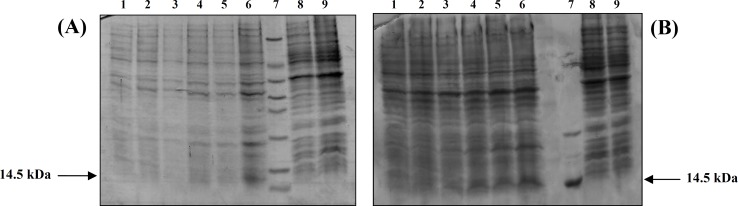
Comparison of SDS-PAGE analysis of mature and mutated recombinant CTB in *E. coli*. A) SDS-PAGE analysis of expression of mature recombinant CTB in *E. coli* M15 (pREP4) using pQE_*ctx*B as expression vector. Lane 1, M15 (pREP4) containing pQE_*ctx*B before induction with IPTG; lane 2-6, indicate 1-5 hours after induction respectively; lane 7, protein size marker and lane 8 and 9, M15 (pREP4) containing pQE used as negative control. B) SDS-PAGE analysis of expression of mutant recombinant CTB in *E. coli* M15 (pREP4using pQE containing mutant *ctx*B gene as expression vector. Lane 1, M15 (pREP4) containing pQE_*ctx*B before induction with IPTG; lane 2-6, indicate 1-5 hours after induction respectively; lane 7, commercial CT (cholera toxin) used as positive control and lane 8 and 9, M15 (pREP4) containing pQE used as negative control
